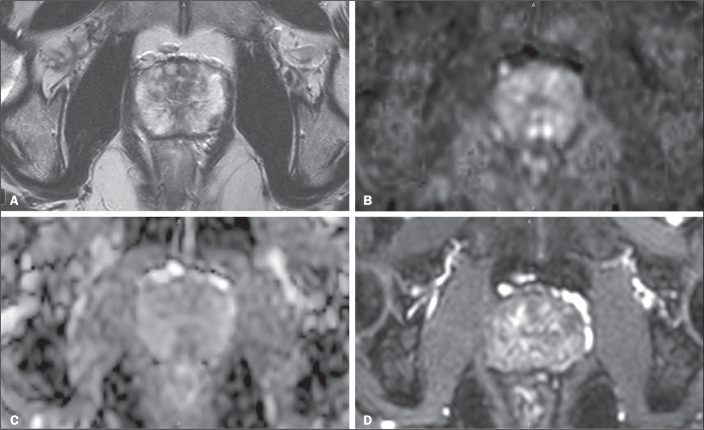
Multiparametric prostate MRI from a 62-year-old patient with suspected
